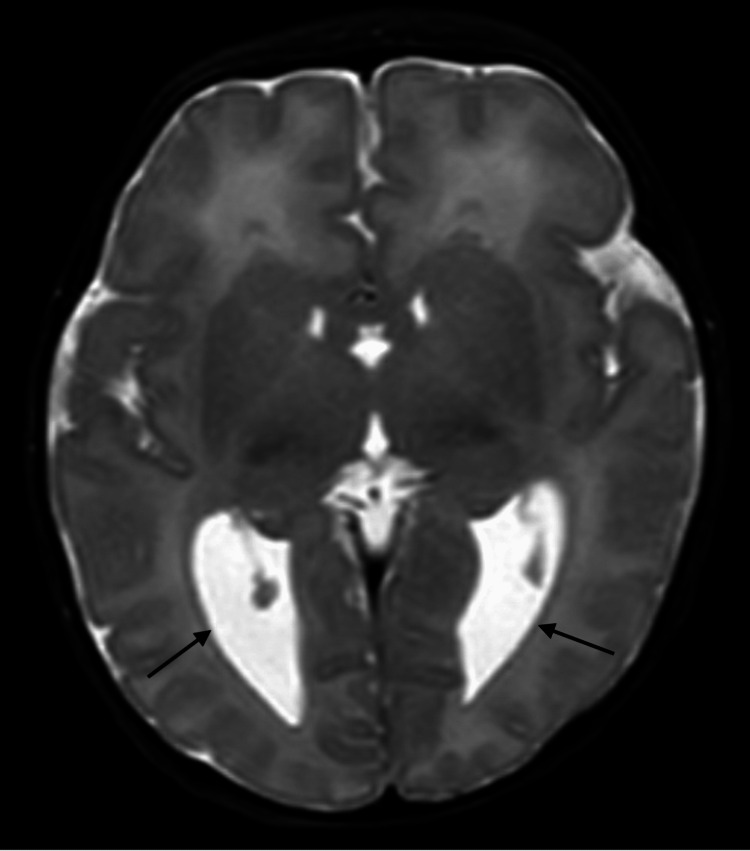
MRI of the brain, T2 sequence in the axial plane, displaying colpocephaly. Source: WellSpan York Hospital.
